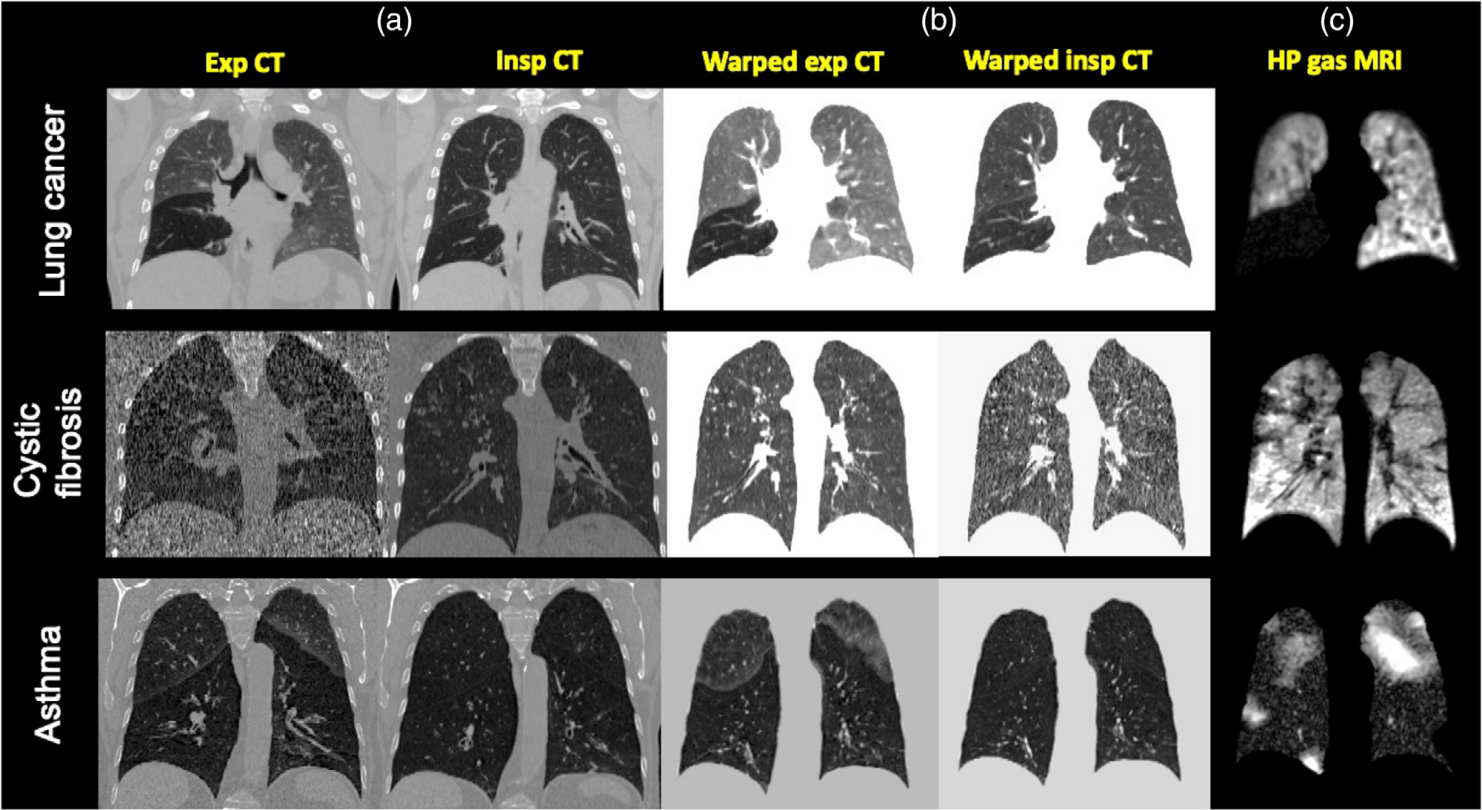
Example coronal slices for three patients with lung cancer, cystic fibrosis, or asthma of inspiratory and expiratory CT scans (a) before and (b) after deformable registration to the spatial domain of (c) hyperpolarized gas MRI.
